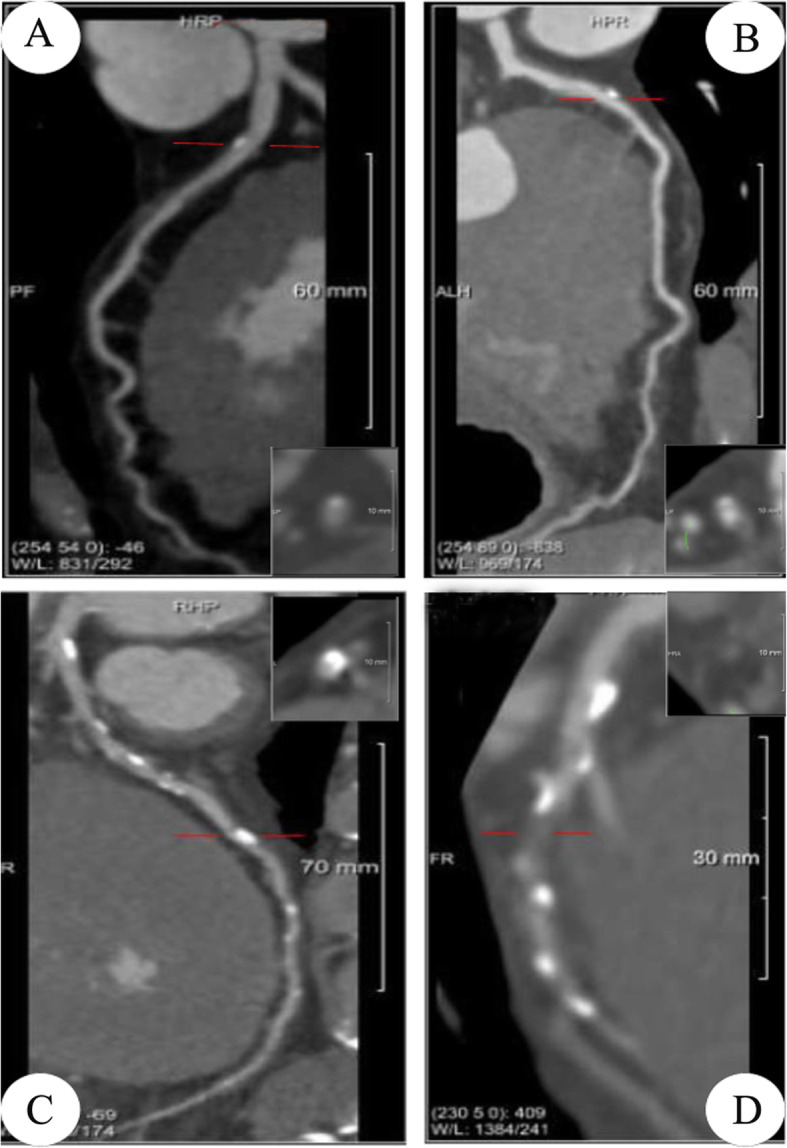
The percentage of left anterior descending coronary artery (LAD) stenosis defined by coronary CT angiography. The degrees of LAD stenosis were ≤ 25% (a), 26 to 49% (b), 50 to 74%(c) and ≥ 75% (d)
